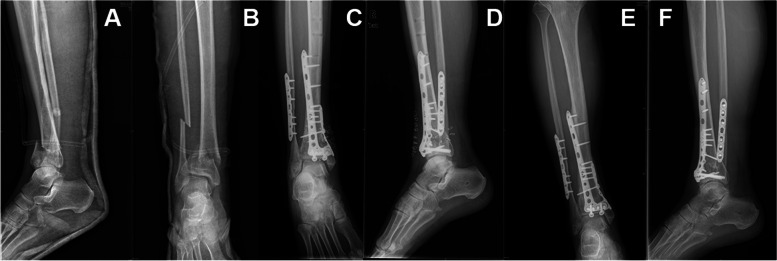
A 55-year-old female with pilon fracture of his left leg from a height fall, Rüedi and Allgöwer II type. A, B Preoperative anteroposterior and lateral radiographs. C, D Anteroposterior and lateral view of postoperative X-ray. E, F Anteroposterior and lateral X-ray 15 months after surgery
